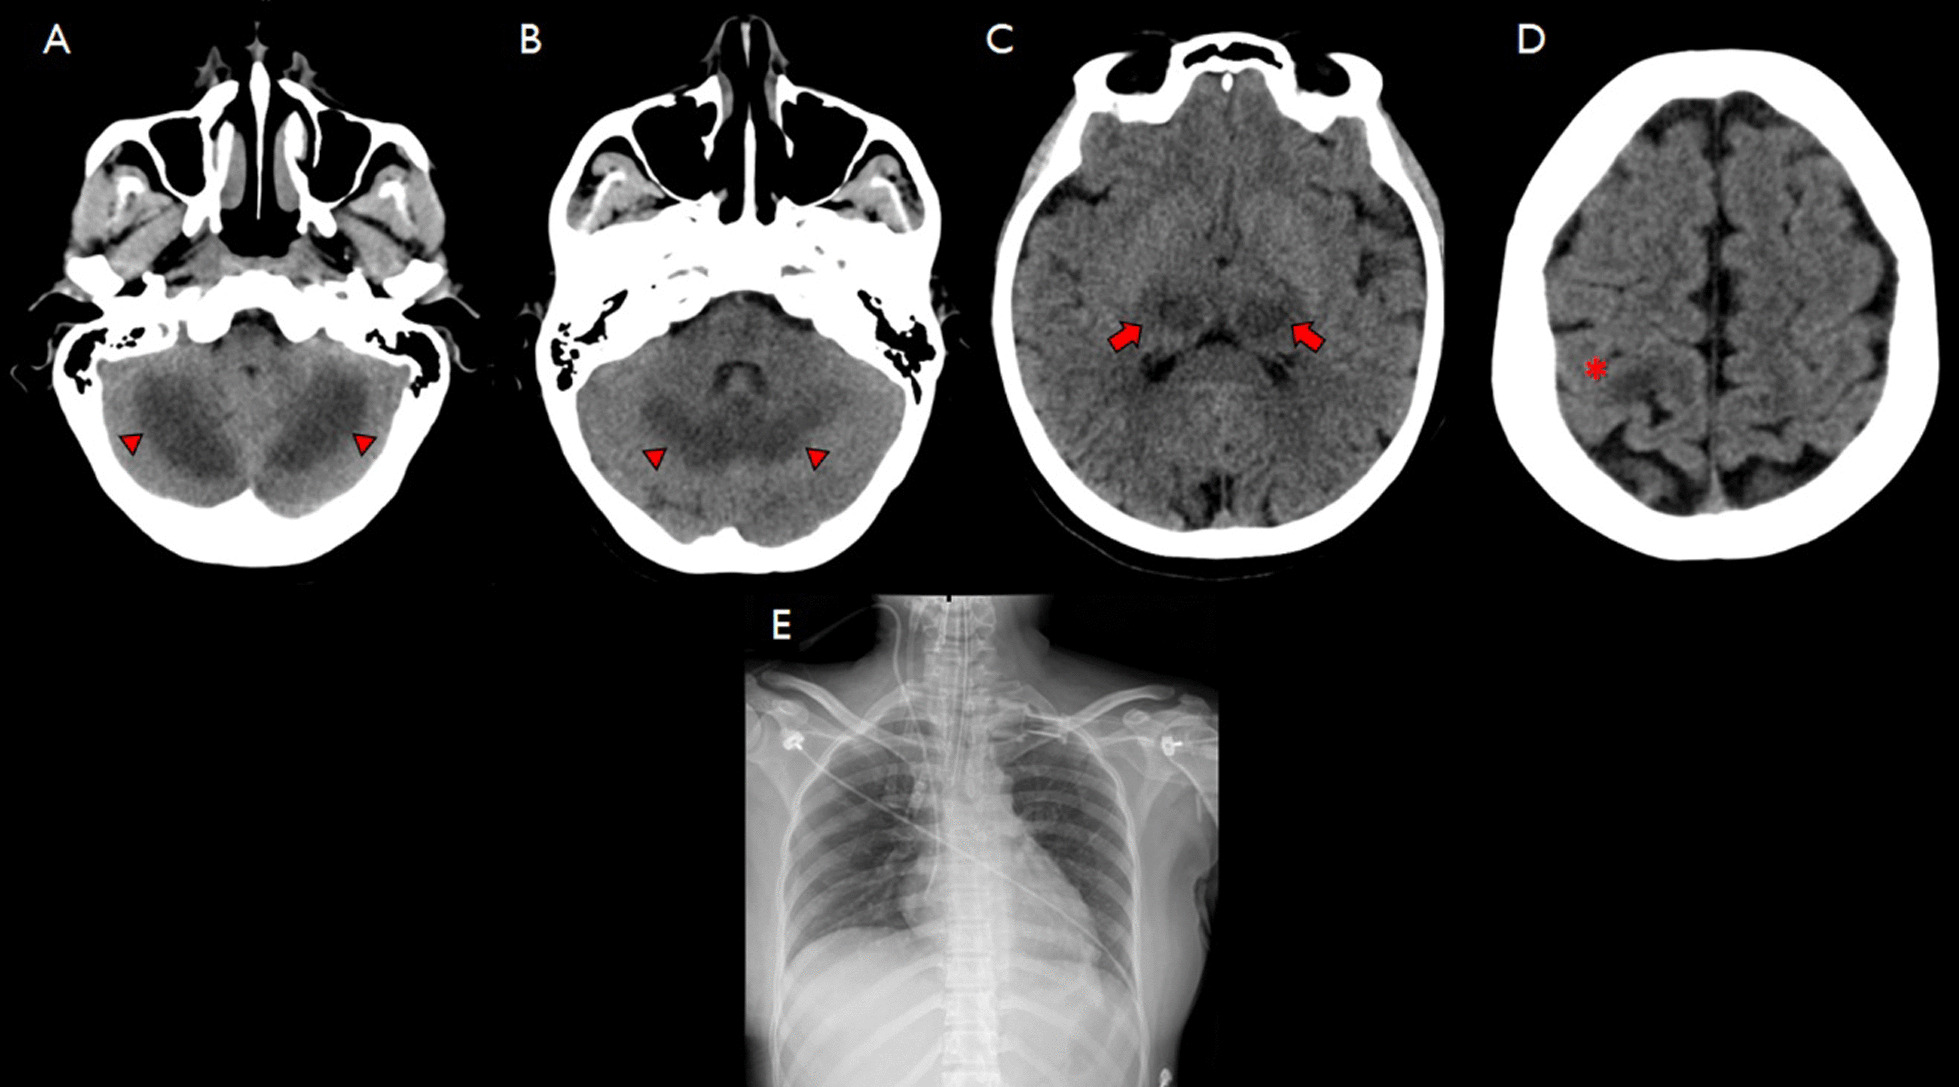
Computerized brain tomography showed bilateral symmetrical hypodense lesions involving thalami (arrows) (C) and cerebellar hemispheres (arrowhead), causing effacement of cerebellar folia (A, B); isolated hypodense lesion at left high frontal area (asterixis) (D). Chest X-ray after intubation showed endotracheal tube tip 0.7 cm above carina, parenchyma was normal with no obvious infiltration (E)
